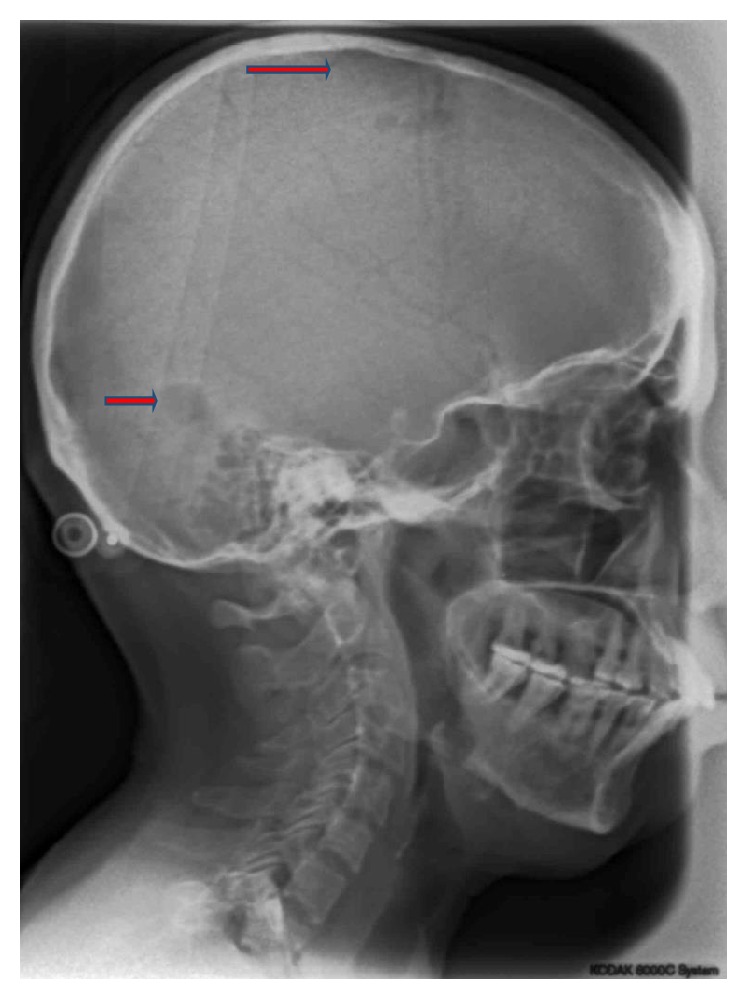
Skull radiograph showing osteolytic areas.
